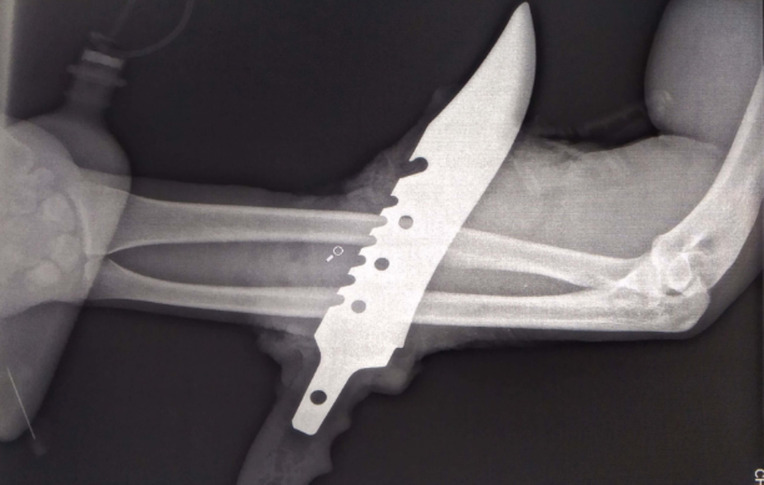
radiographie de l’avant-bras gauche montrant le type de couteau, son siège et sa direction
